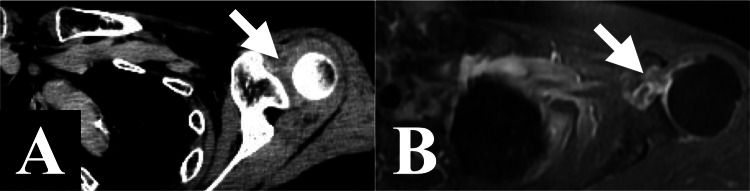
Case 2. Contrast-enhanced CT and MRI findings. (A) Axial CT of CK-SBRT planning showing a contrast-enhanced lesion at the rotator interval of the left shoulder joint. (B) Just before CK-SBRT, axial MRI showing a contrast-enhanced lesion at the rotator interval of the left shoulder joint. CT: computed tomography; MRI: magnetic resonance imaging; CK-SBRT: stereotactic body radiotherapy using CyberKnife
